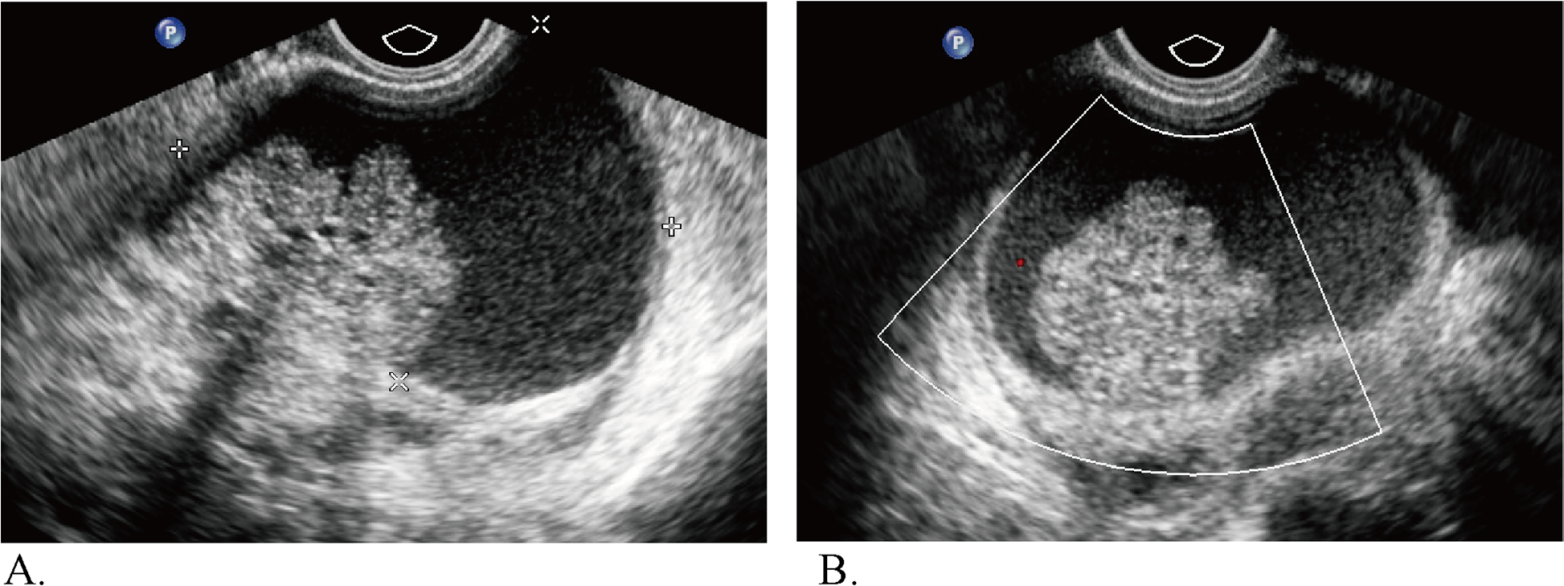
Ultrasound images of a 41-year-old woman with mucinous adenofibroma. The level of CA 125 was 210 U/ml. A Transvaginal ultrasound revealed a 7.3-cm complex cystic lesion with a solid component > 7 mm in the right adnexa. B Color Doppler ultrasound revealed no color flow (color score = 1). The lesion was categorized as IOTA SR inconclusive and O-RADS 4, according to US category systems
